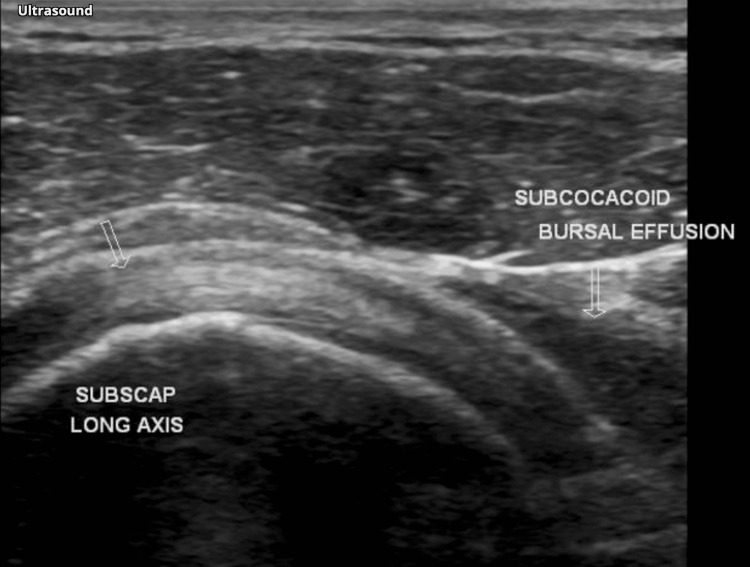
Transverse ultrasound of the shoulder joint revealed complete tears in both the subscapularis and supraspinatus tendons as indicated by an arrowhead Image source: Ref. [11].
